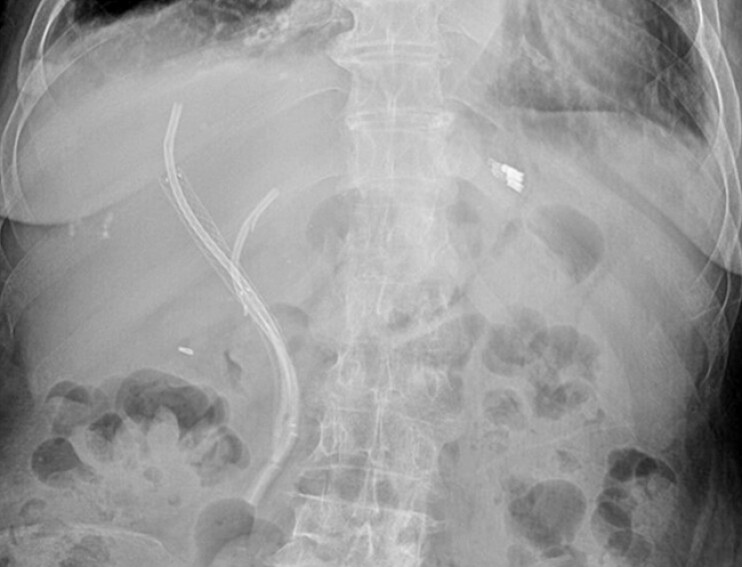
Abdominal plain film imaging after ERCP. Abdominal X-ray showing two 8.5-French plastic stents in situ within the left and right hepatic ducts. ERCP, ERCP, endoscopic retrograde cholangiopancreatography.
